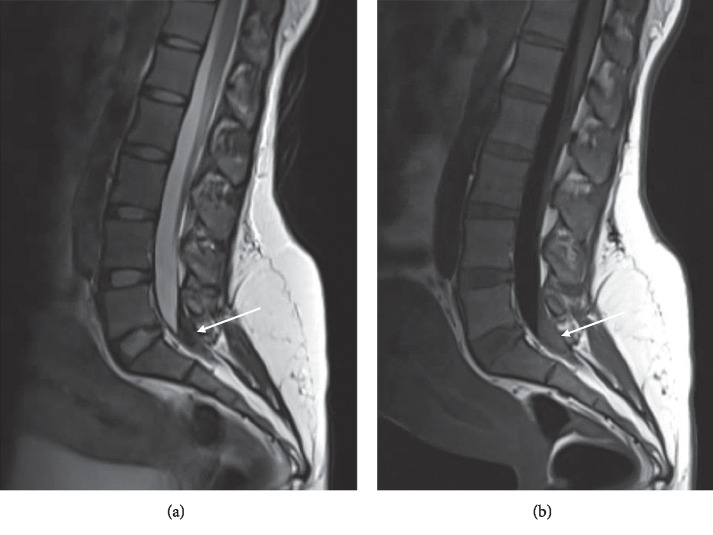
Sagittal T2-weighted Dixon fat saturated (a) and T1-weighted FLAIR (b) images showing blood collection within the thecal sac extending from the level of the L4-L5 decubitus base down to the level of S1-S2. This is most pronounced at the level of L5-S1 where the hematoma occupies the entire thecal sac and surrounds the cauda equina (arrow).
